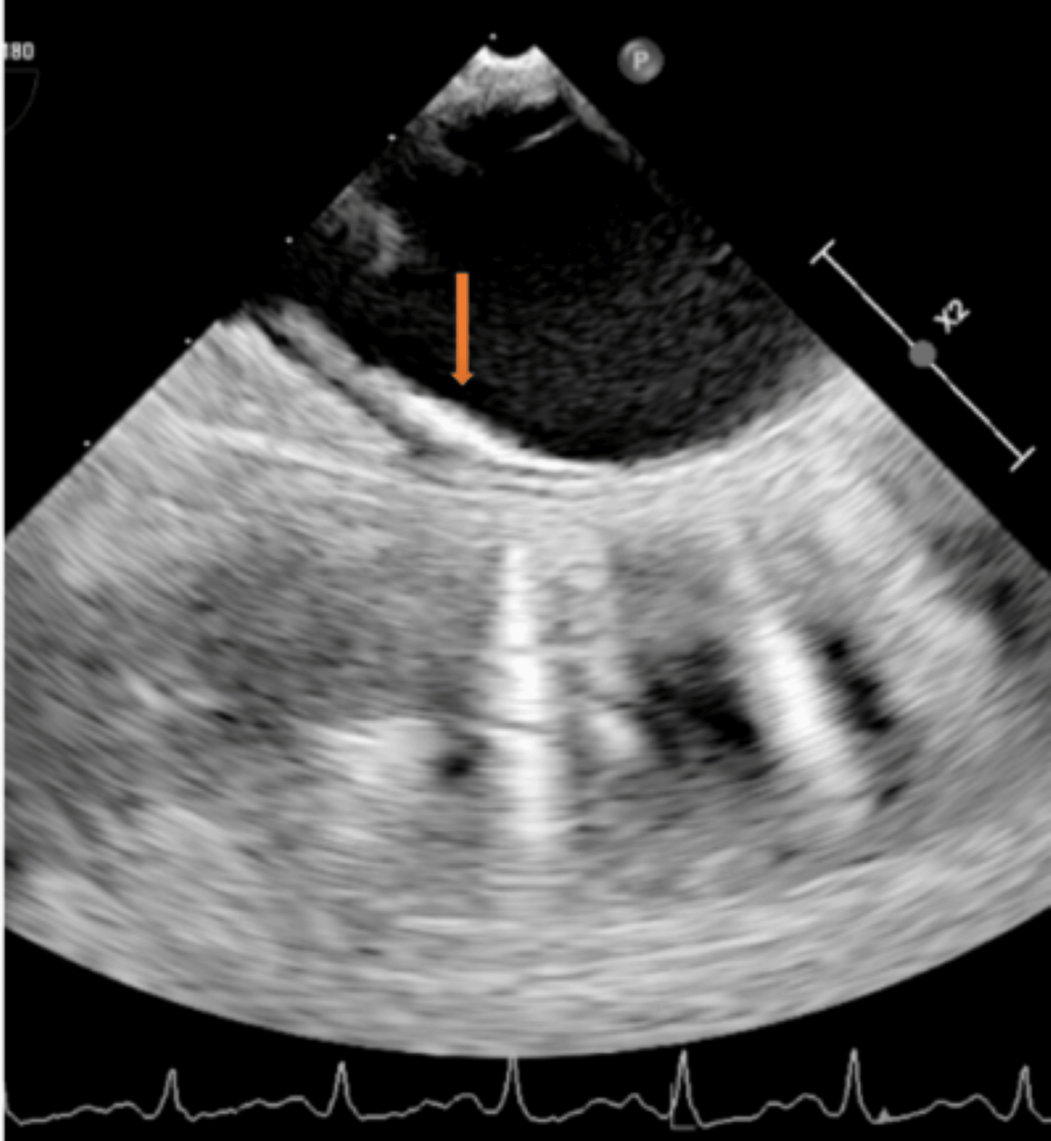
Atherosclerotic plaque in the aorta X2 denotes a two-fold zoom applied by the echocardiography machine, indicating image magnification rather than an anatomical distance measurement.
